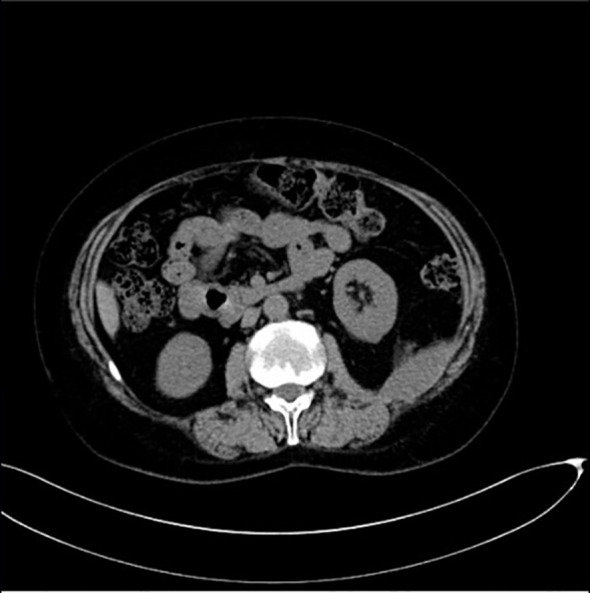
The follow-up CT demonstrates significant resolution of the hematoma.
